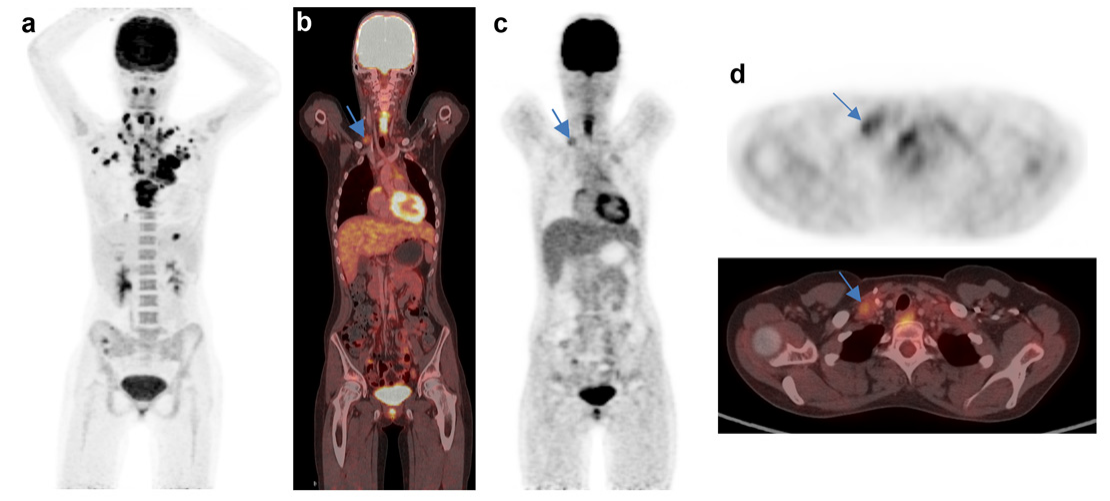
Discrepant D scoring of the right supraclavicular region. Case 2.: a) extensive lymphoma involvement including the right supraclavicular region before treatment. b—d) enhanced FDG uptake in a right supraclavicular node clearly exceeding that of the mediastinum. It is difficult to decide if the nodal uptake is higher or less than the liver uptake. Deauville score of the readers R1-5 for the supraclavicular region, respectively: 3-4-3-3-4. Courtesy Dept. Nuclear Medicine, University Hospital Erlangen, Germany.
